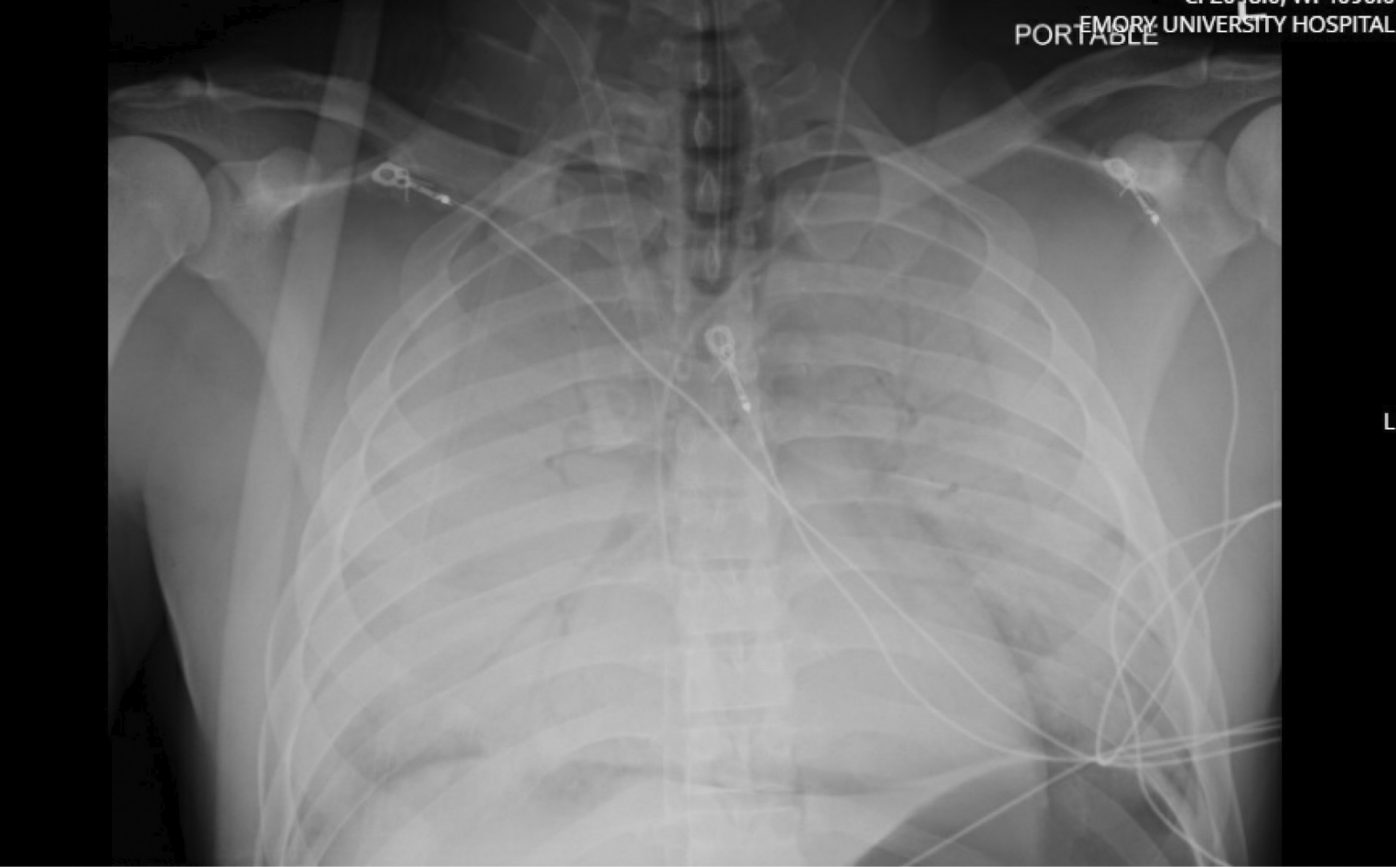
Portable chest radiograph from hospital day 1. This radiograph demonstrates complete opacification of bilateral lung fields with appropriately positioned ECMO cannulas 8.3 cm apart.
